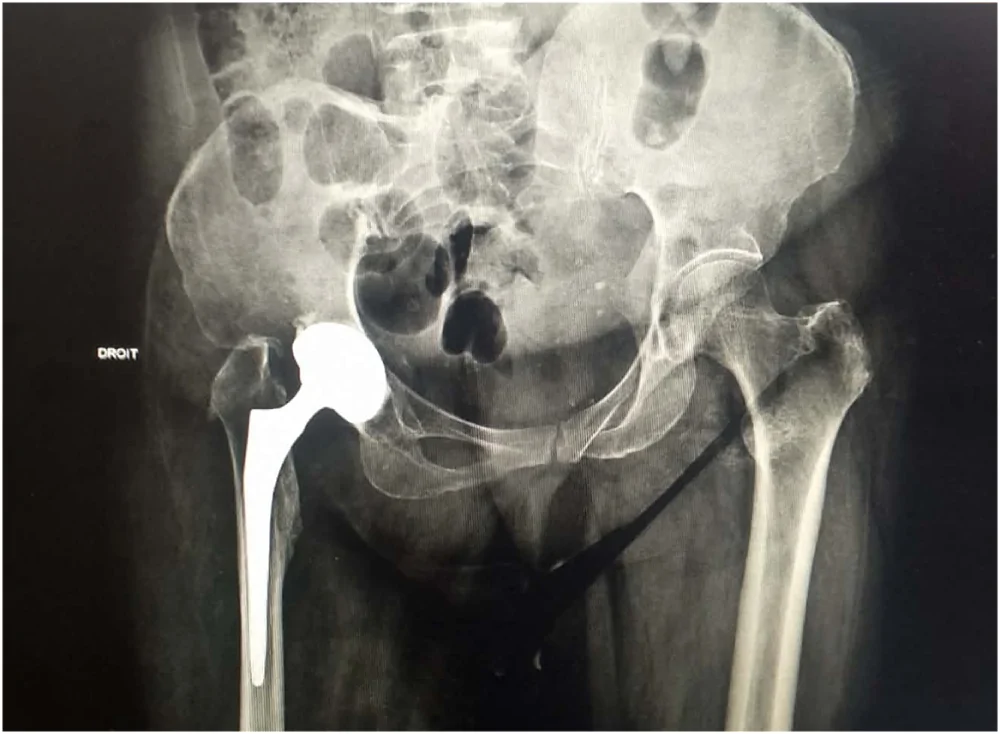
Postoperative anteroposterior pelvic radiograph showing good implant positioning and integration.
